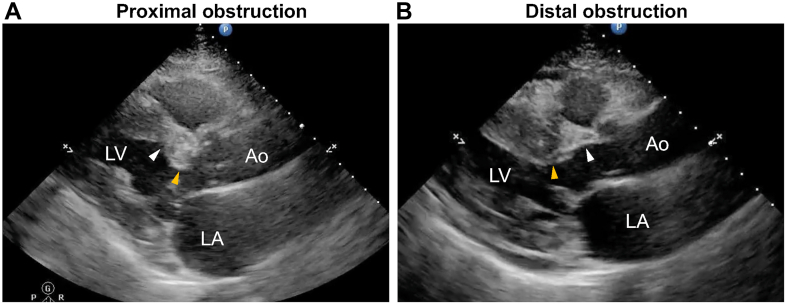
Mechanisms of Residual Obstruction at the Left Ventricular Outflow Tract (A) Representative echocardiographic image of proximal obstruction. The previously ablated myocardium (white arrowhead) shows regression with no LV obstruction, whereas systolic anterior motion of the mitral leaflet occurs at the level of the uncovered myocardium (orange arrowhead) proximal to the previous ablation site, resulting in residual proximal obstruction. (B) Representative echocardiographic image of distal obstruction. The previously ablated myocardium (white arrowhead) shows regression with no LV obstruction, whereas systolic anterior motion of the mitral leaflet occurs at the level of the uncovered myocardium (orange arrowhead) distal to the previous ablation site, resulting in residual distal obstruction. Ao = Aorta; LA = left atrium; LV = left ventricle.2)*Distal obstruction*: residual basal-to-mid LV obstruction at the mitral leaflet coaptation level distal to the previous ablation site (Figure 1B, Video 2).
